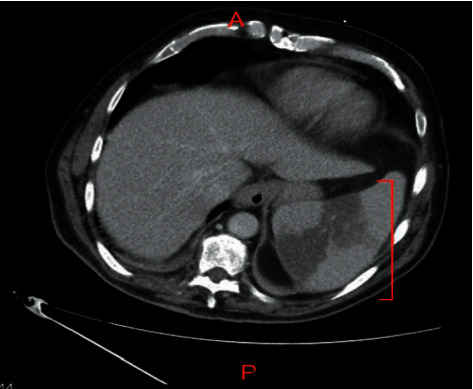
Axial CT abdomen and pelvis imaging showing an enlarged spleen with evidence of acute segmental infarction with well-defined hypodensities.
